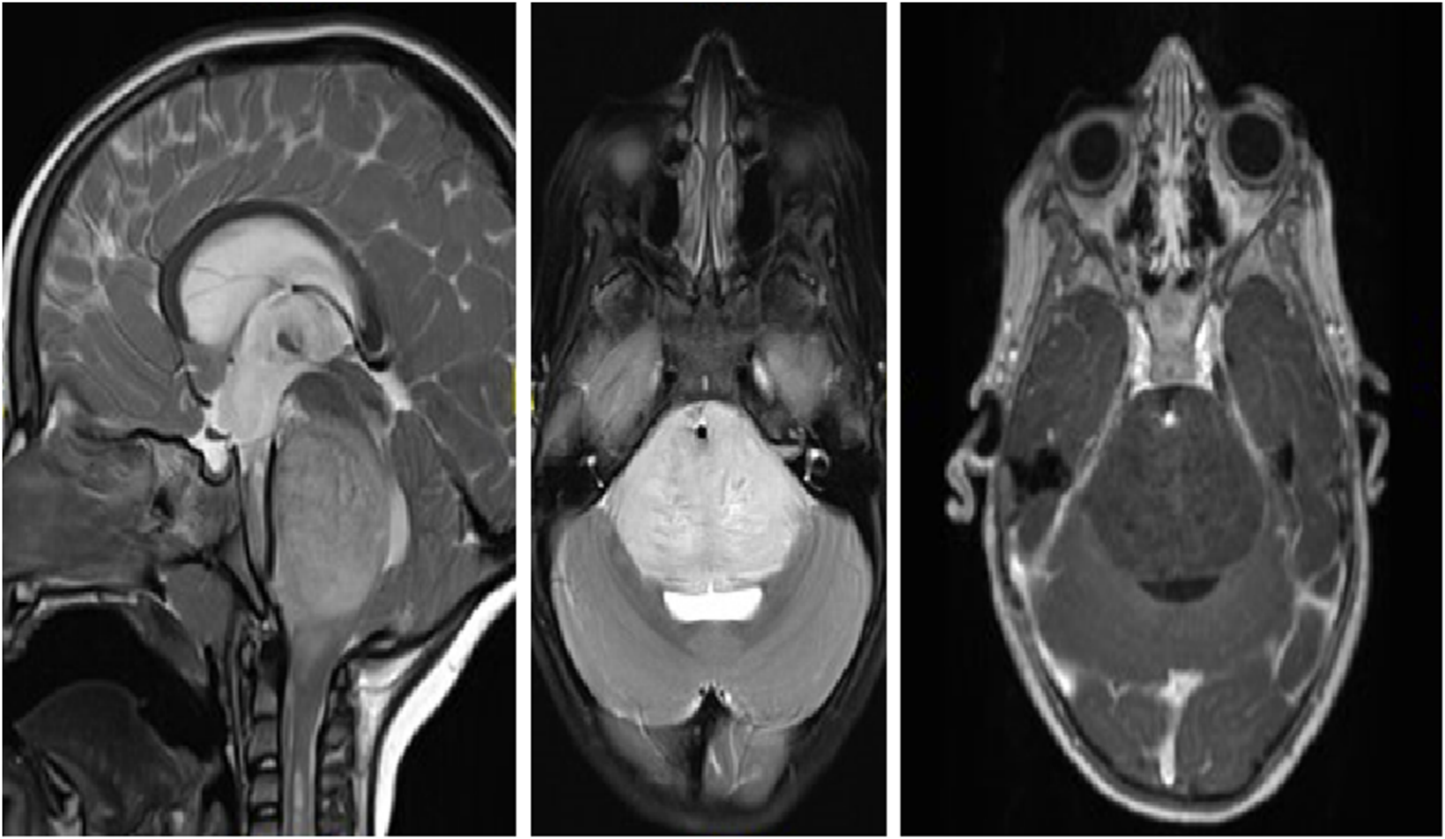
Diffuse intrinsic pontine glioma (DIPG): sagittal (left) and axial (middle) T2-weighted, and axial (right) T1-contrast-enhanced MRI of a 3.5-year-old girl presenting with new-onset cranial neuropathy, motor decline, and headaches. MRI shows a diffuse pontine tumor, with engulfment of the basilar artery, and very mild linear contrast enhancement. A shunt was placed to treat the hydrocephalus. Based on the MRI features, a DIPG was suspected and treated accordingly
